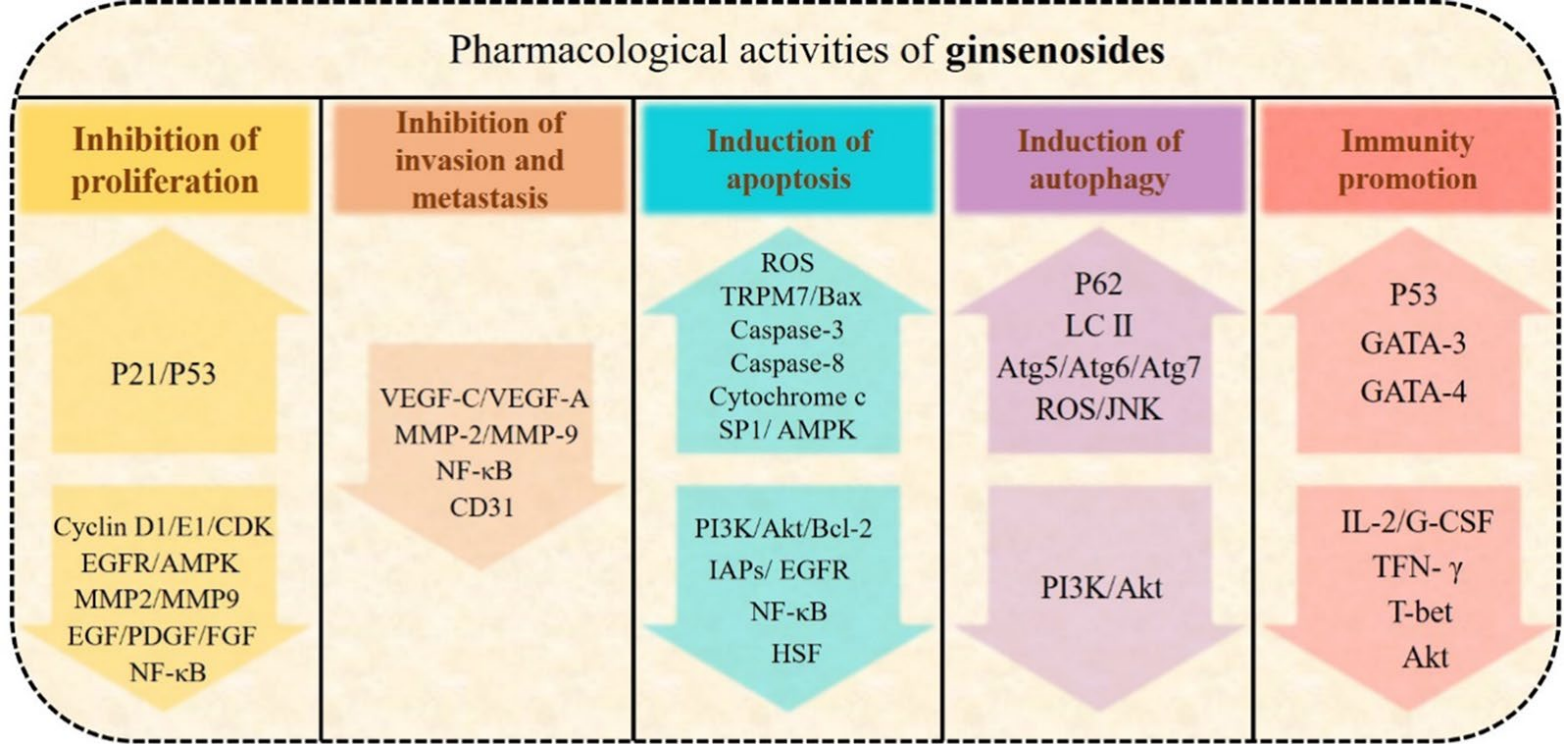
Anticancer activities of ginsenosides. The arrow upward in the figure indicates the upregulation of gene expression; the arrow downward indicates the downregulation of gene expression. *P21* cyclin-dependent kinase inhibitor, *P53* tumor suppressor and transcription factor, *CDKs* cyclin-dependent kinases, *EGFR* epidermal growth factor receptor, *AMPK* 5 AMP-activated protein kinase, *MMP* matrix metalloproteinase, *EGF* epithelial growth factor, *FDGF* platelet derived growth factor, *FGF* fibroblast growth factor, *NF-κB* nuclear factor κB, *VEGF* vascular endothelial growth factor, *CD31* Platelet endothelial cell adhesion molecule-1, *ROS* reactive oxygen species, *TRPM7* transient receptor potential melastatin 7, *Bax* bcl2 associated X protein, *SP1* transcription factor Sp1, *PI3K* phosphatidylinositol 3-kinase, *Akt* protein kinase B, *Bcl-2* B-cell lymphoma-2, *IAPs* inhibitor of apoptosis proteins, *EGFR* epidermal growth factor receptor, *HSF* the heat shock factor, *P62* sequestosome 1, *LC3-II* the processed form microtubule-associated protein 1 light chain 3, *Atg* autophagy-related protein, *JNK* c-Jun NH2-terminal kinase, *GATA* T cell specific transcription factor, *IL-2* Interleukin-2, *G-CSF* granulocyte colony-stimulating factor, *TNF* tumor necrosis factor, *T-bet* T-box transcription factor
